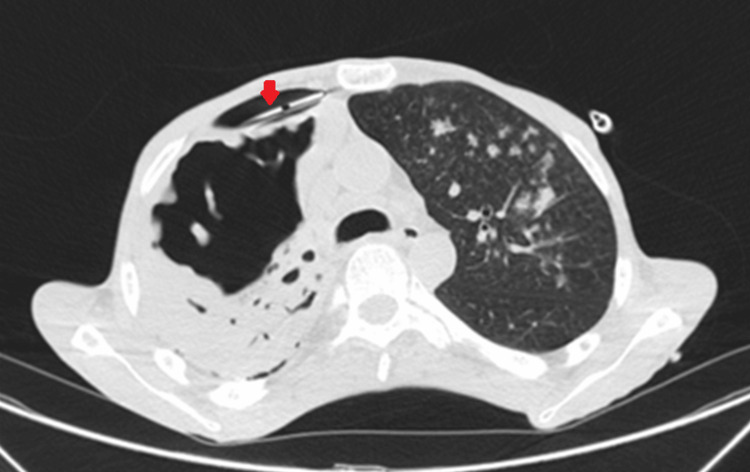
Axial chest CT scan image Axial image showing correct chest tube placement (red arrow) with persistent pneumothorax.
